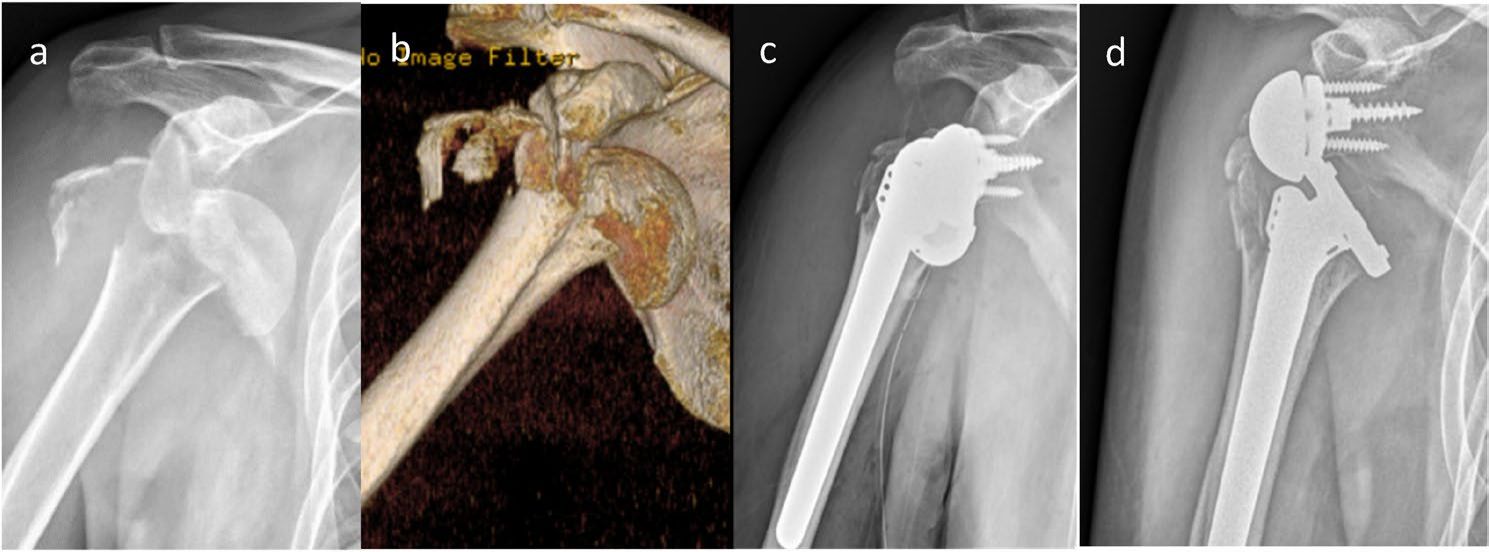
Case 1. Right proximal humerus fracture in a 69-year-old male. a X-ray. b CT scan. c RTSA after fracture at first time. d Dislocation occurring 2 weeks post-surgery
